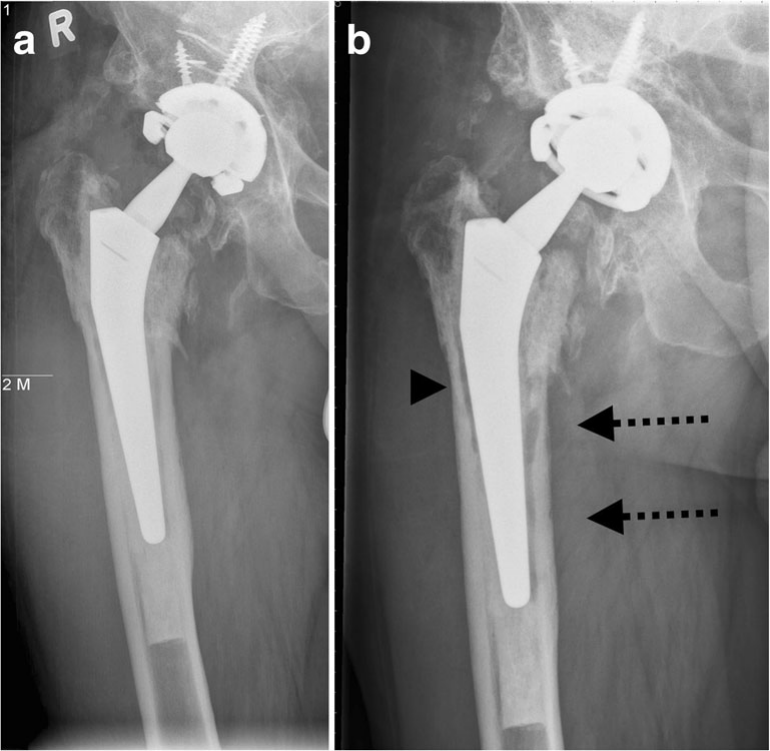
a AP radiograph, 4 months postoperatively. Cemented (hybrid) total hip arthroplasty after revision with acetabular fixation screws. Normal postoperative findings. b AP radiograph of the same patient, 5 months postoperatively. Periprosthetic lucencies in Gruen zone 5/6 (*arrows*) and more discrete in Gruen zone 2 (*arrowhead*): proven case of infection
